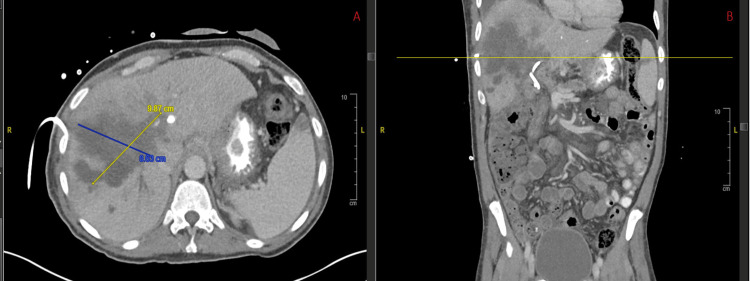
Contrast-enhanced CT scan of the abdomen. (A) Axial view showing altered perfusion of the right hepatic lobe with multiple hepatic metastases, segmental intrahepatic biliary dilation, and cystic lesions suggestive of biliary cysts or infected bilomas. (B) Coronal view demonstrating progression of hepatic metastases, near-occlusion of portal vein branches, and associated intrahepatic cystic changes
